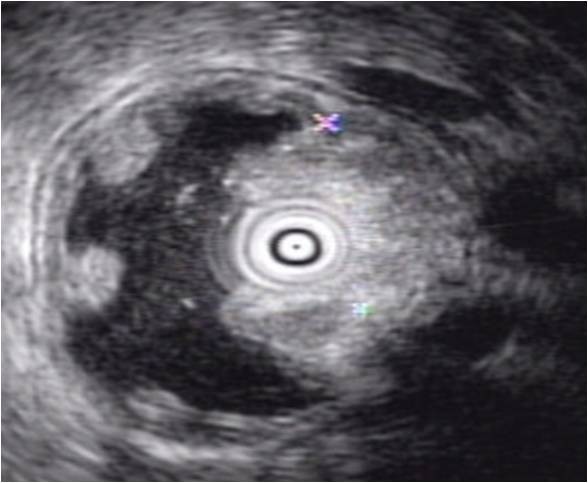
Endoscopic ultrasonography finding. There was 1.3 cm sized ill defined hypoechoic mass in submucosal layer at minor papilla.
